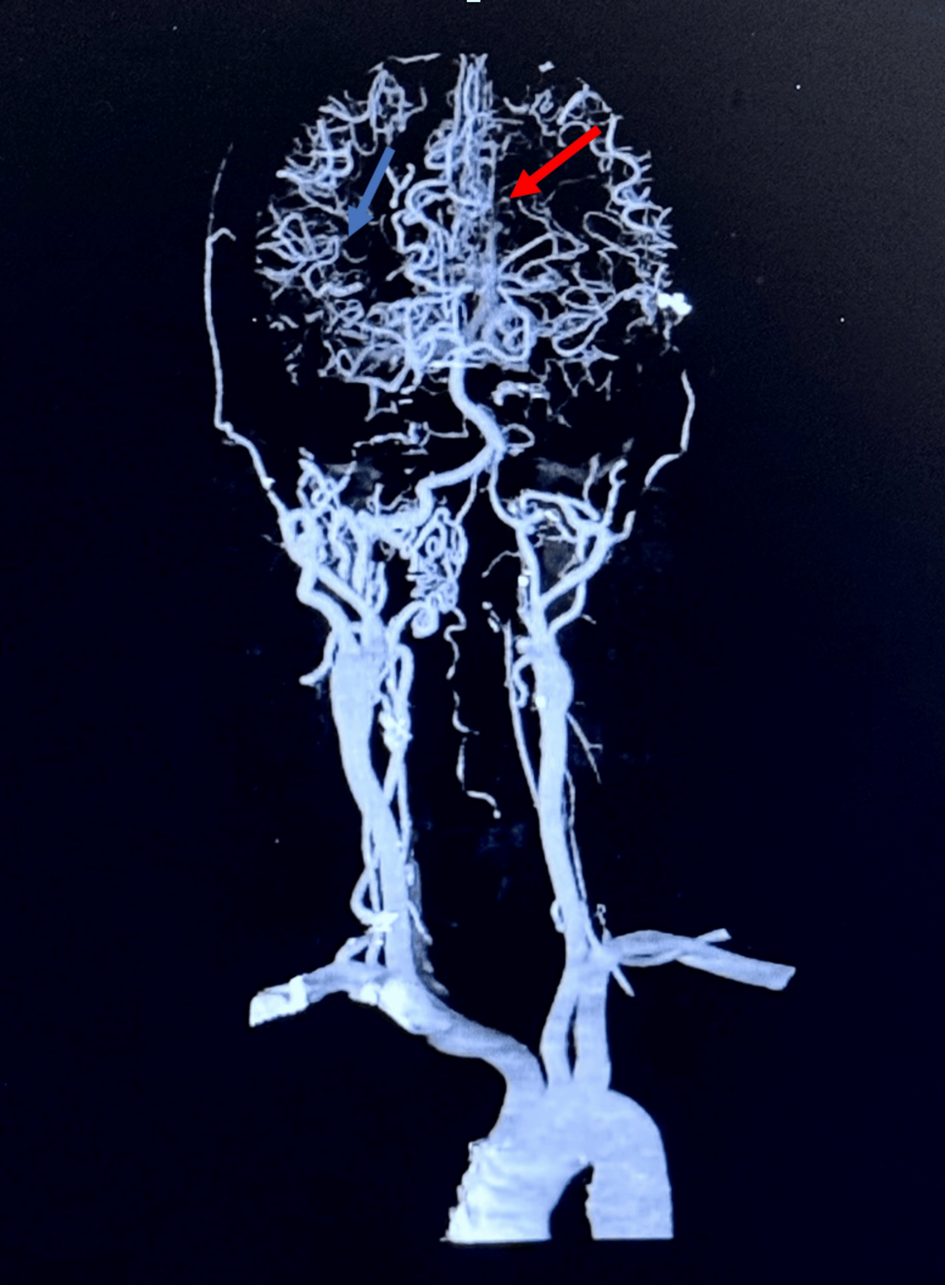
CTA of head and neck The red arrow highlights the "puff of smoke" or moyamoya pattern, with abnormal dilated collateral vessels forming in response to stenosis/occlusion of major cerebral arteries from the combined effects of sickle cell disease and moyamoya phenomenon. The blue arrow indicates the abrupt termination or cut-off of the distal right M3 segment of the middle cerebral artery due to vaso-occlusive changes from sickle cell disease. CTA: computed tomography angiography
